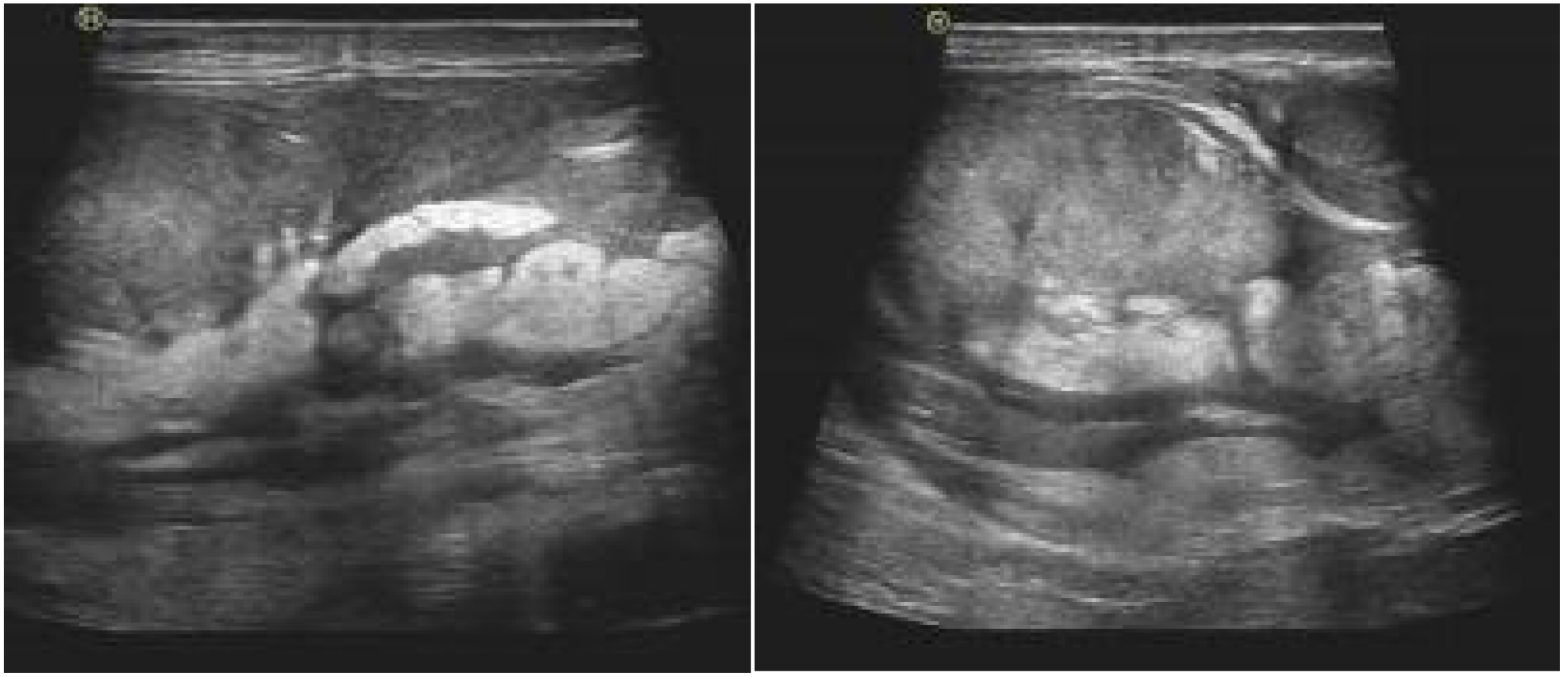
Ultrasound examination showed uneven echo quality in the sperm area above the left testicle. The mass is approximately 113 x 83 mm, with unclear limitations, irregular morphology, internal echo inequality and fat-like echoes extending to the inguinal canal.
